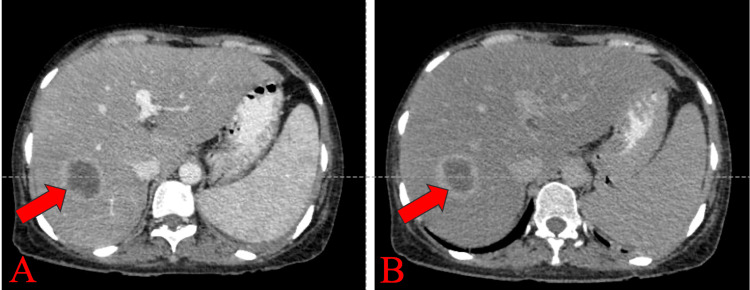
CECT-AP axial section: (A) venous phase showing a well-defined hypodense lesion with peripheral enhancement in segments VII and IV of the liver (shown by the red arrow) likely to be a hepatic abscess and (B) delayed phase showing the same lesion with peripheral enhancement shown by the red arrow. CECT-AP: contrast-enhanced computed tomography of the abdomen and pelvis
